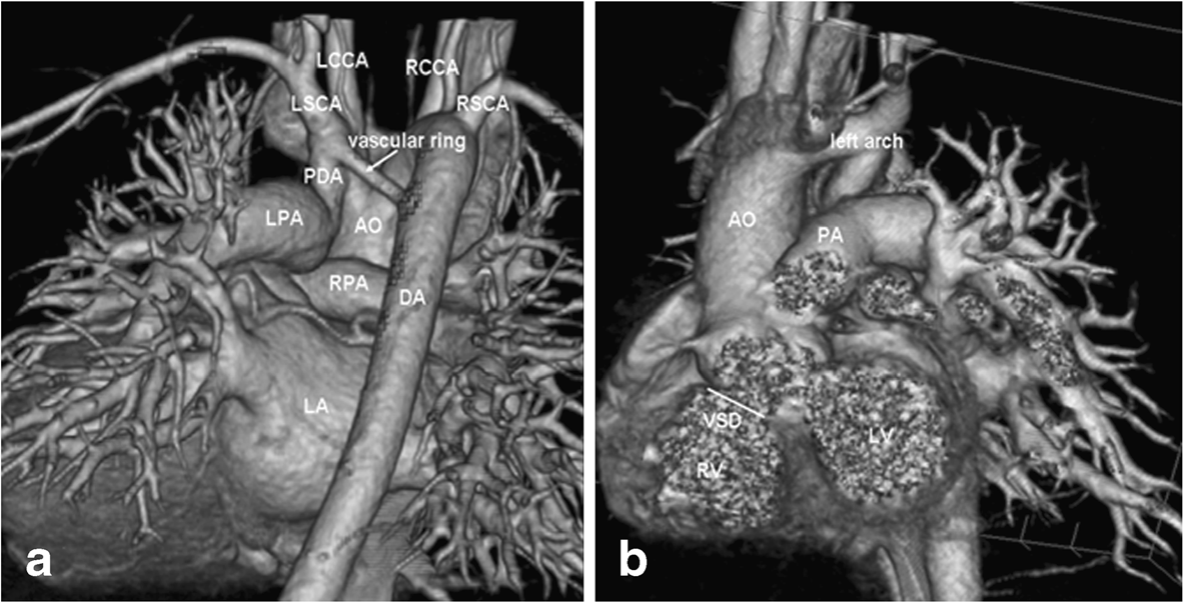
3D-volume rendered image of the contrast enhanced multi-slice CT (a) in posteroanterior and (b) in anteroposterior. AO = aorta, DA = descending aorta, PDA = patent ductus arteriosus, LPA = left pulmonary artery, RPA = right pulmonary artery, LCCA = left common carotid artery, LSCA = left subclavian artery, RCCA = right common carotid artery, RSCA = right subclavian artery, RV = right ventricle, LV = left ventricle, VSD = ventricular septal defect, LA = left atrium.
